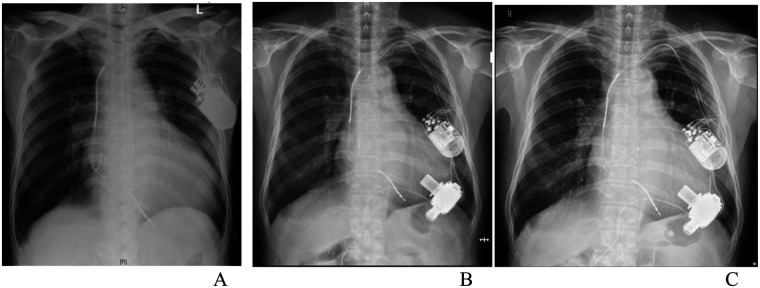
Changes in chest radiographs. (A) At the time of admission, the cardiac shadow was enlarged. After the implantation of a cardiac resynchronization therapy defibrillator, the pulmonary congestion was improved. (B) On 24 February 2023 (POY2.5), the cardiac shadow was reduced, and the pulmonary congestion was improved. (C) On 21 March 2025 (POY4.5), no significant change in the cardiac silhouette was observed.
